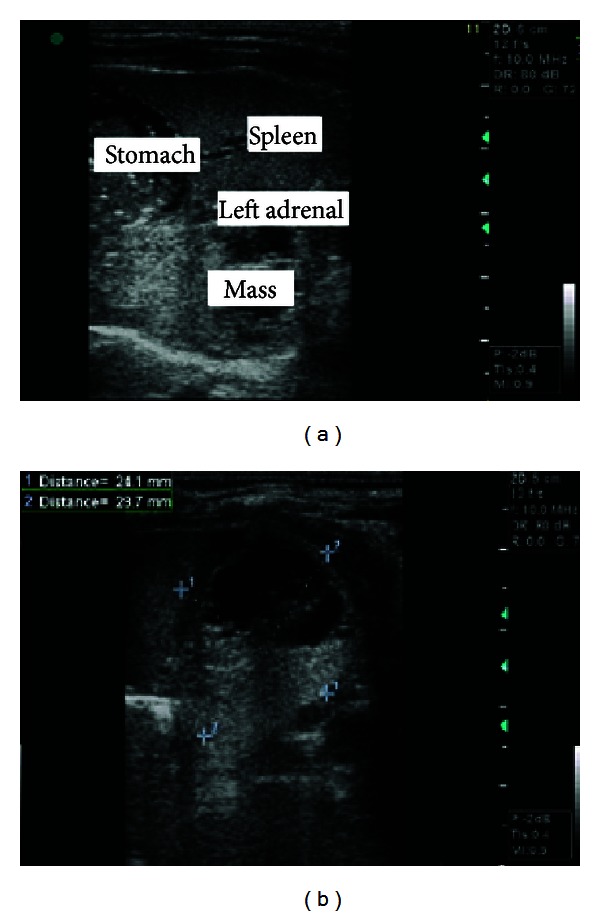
(a) Ultrasonography showing the presence of a retroperitoneal mass, posterior to the left adrenal gland and spleen. (b) Ultrasonography showing the measurements on the mass, of heterogenous appearance with an anechoic formation inside it.
